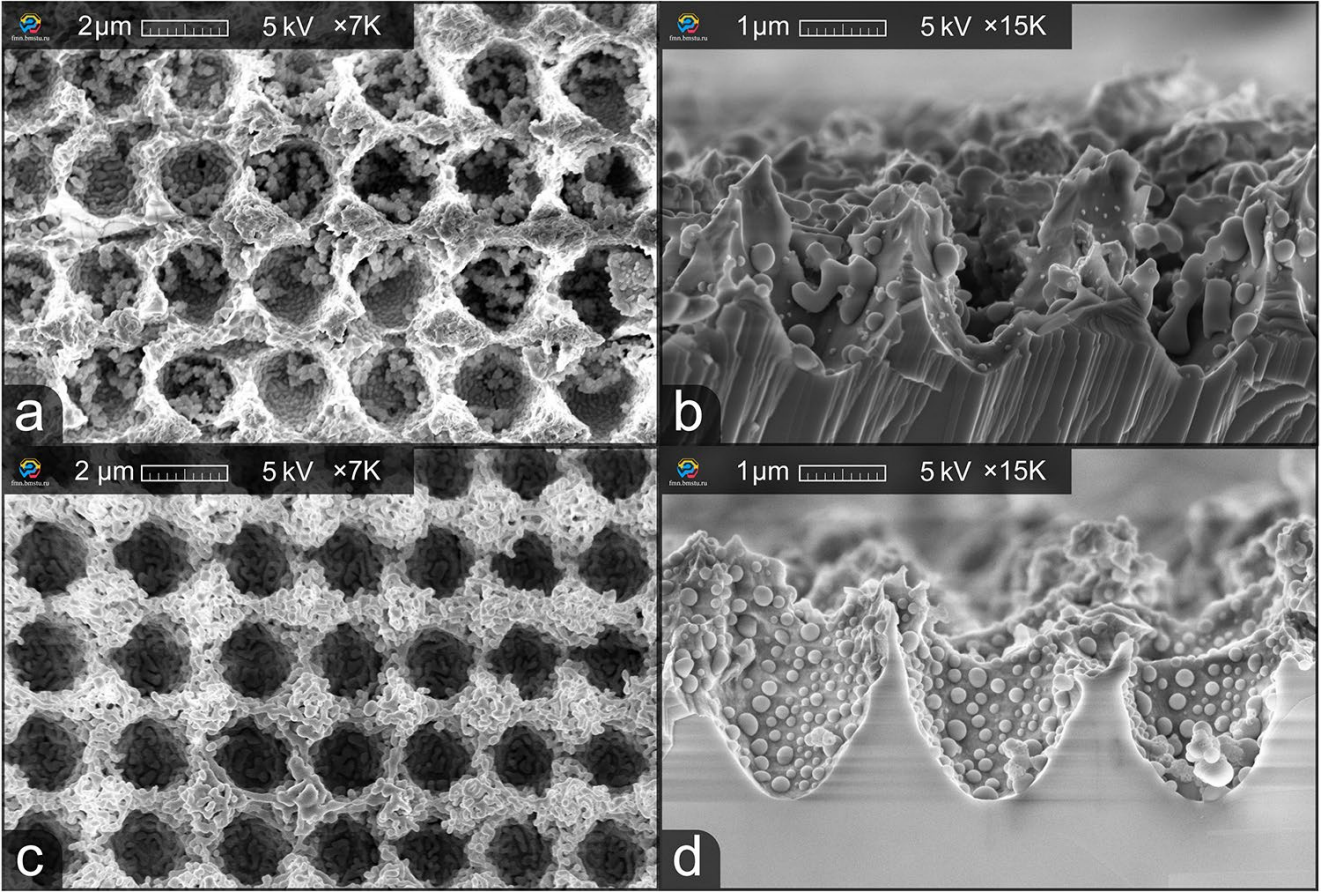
Ultrasonic damage of ARM during etching in solution B and consequent rinsing: top-down (a), cross-section (b); and during rinsing: top-down (c), cross-section (d).
